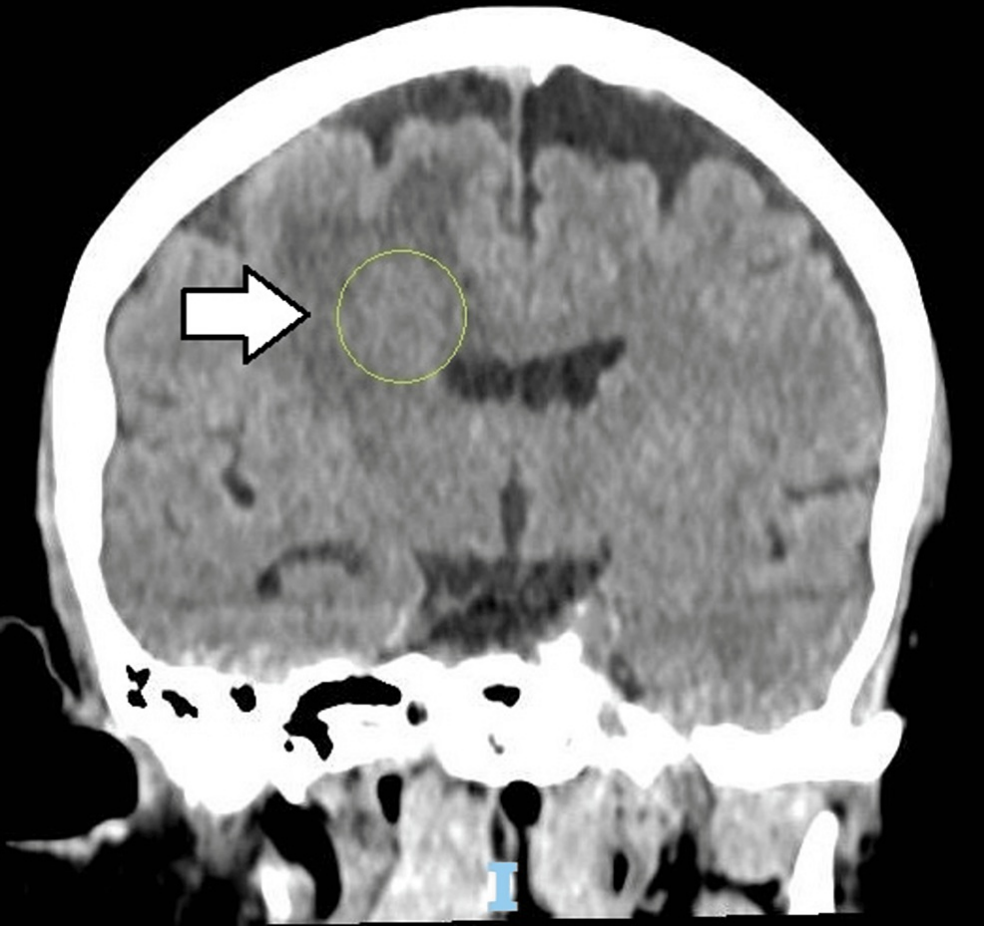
Brain CT scan showing a 1.4x1.3x1.9 cm heterogeneous mass (green circle) at the right frontal lobe (arrow)
